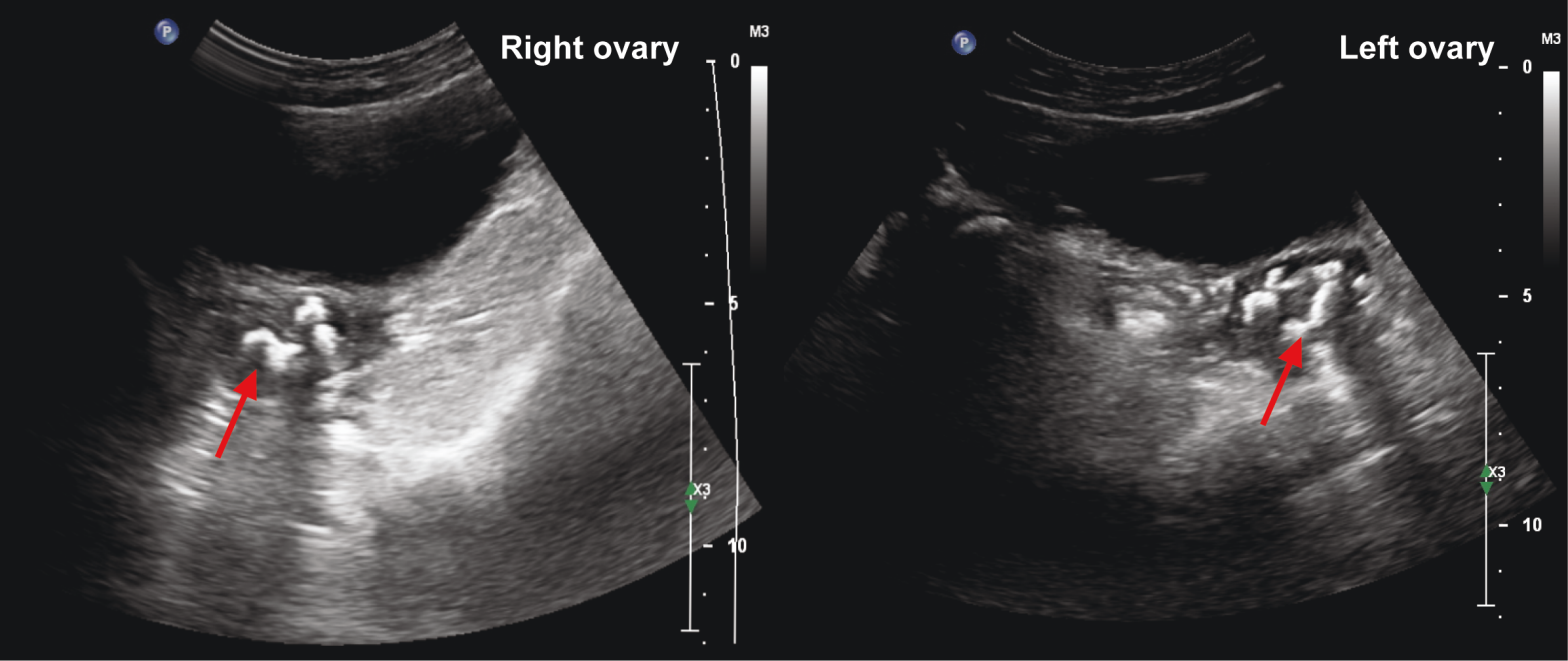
Pelvic ultrasound scan showing areas of calcification (red arrows) in both ovaries.
